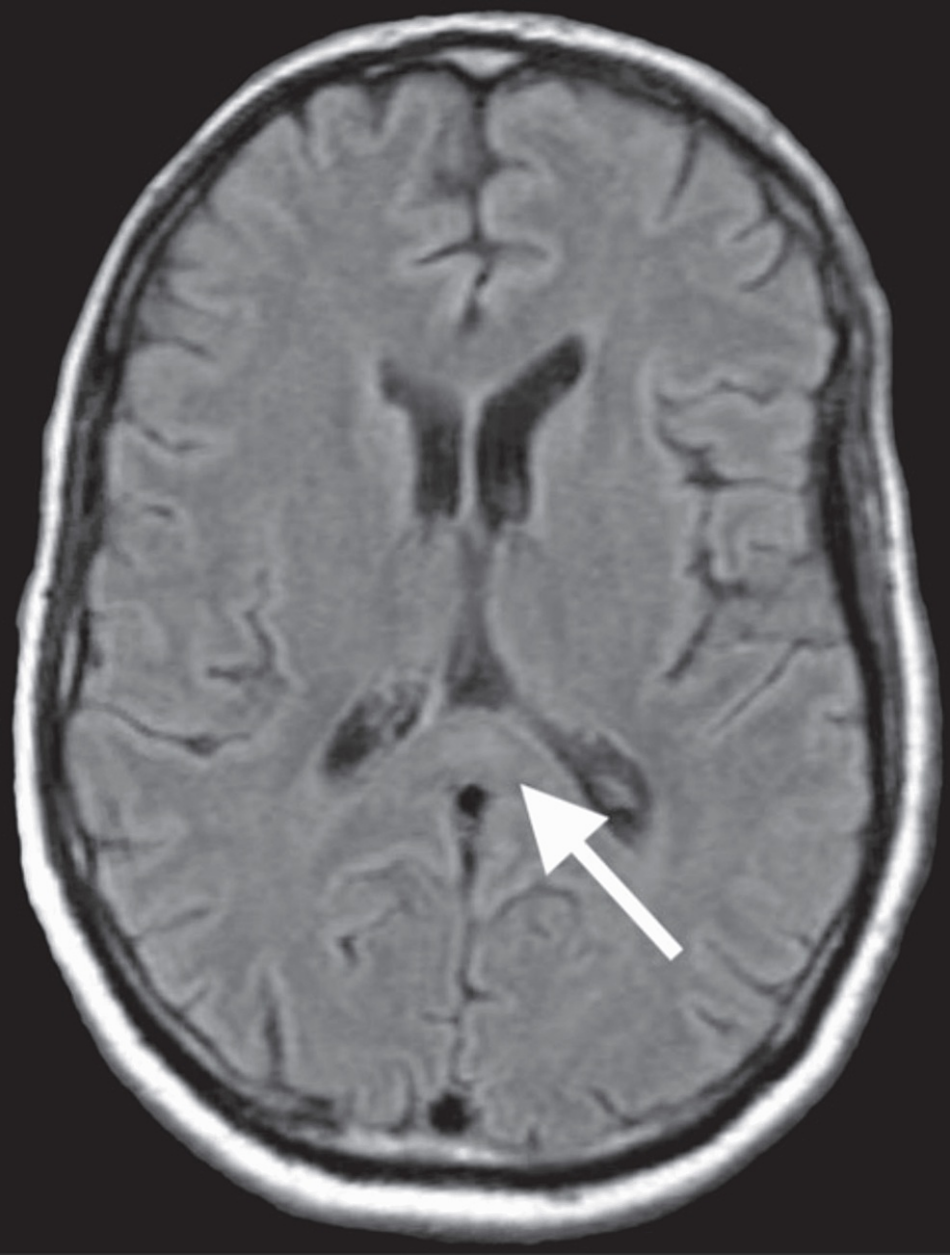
MRI of the brain showing T2/fluid attenuated inversion recovery signal hyperintensity of the splenium of the corpus callosum
